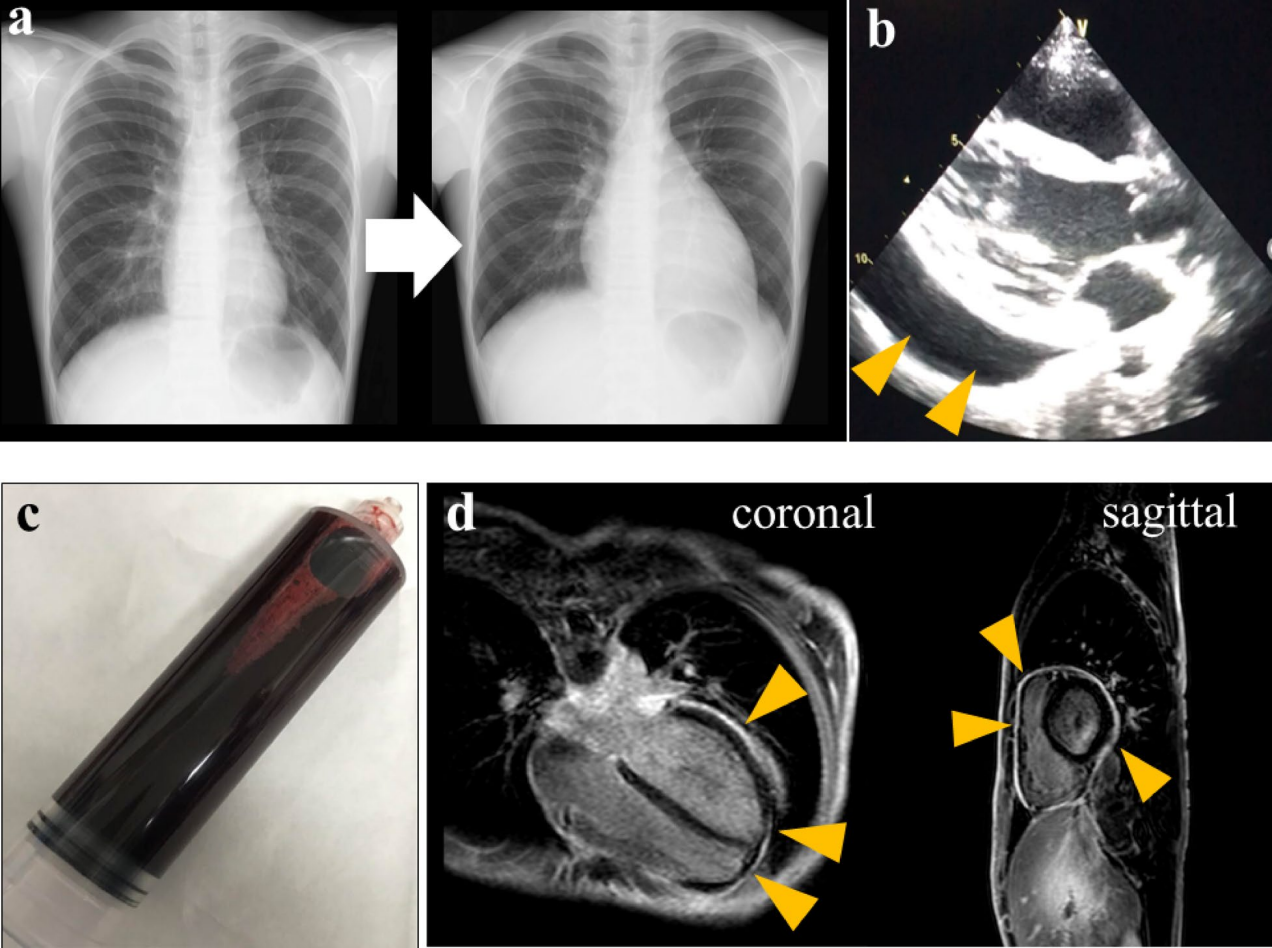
Findings of cardiovascular complications: a Cardiothoracic ratio on chest X-ray changes from 41.9% to 55.0% in a week. b Echocardiography shows a pericardial effusion (arrowhead). c Aspirated content by pericardiocentesis shows the bloody pericardial effusion. d Cardiac MRI shows contrast-enhanced delay consistent with the pericardium (arrowhead), suggestive of pericarditis
